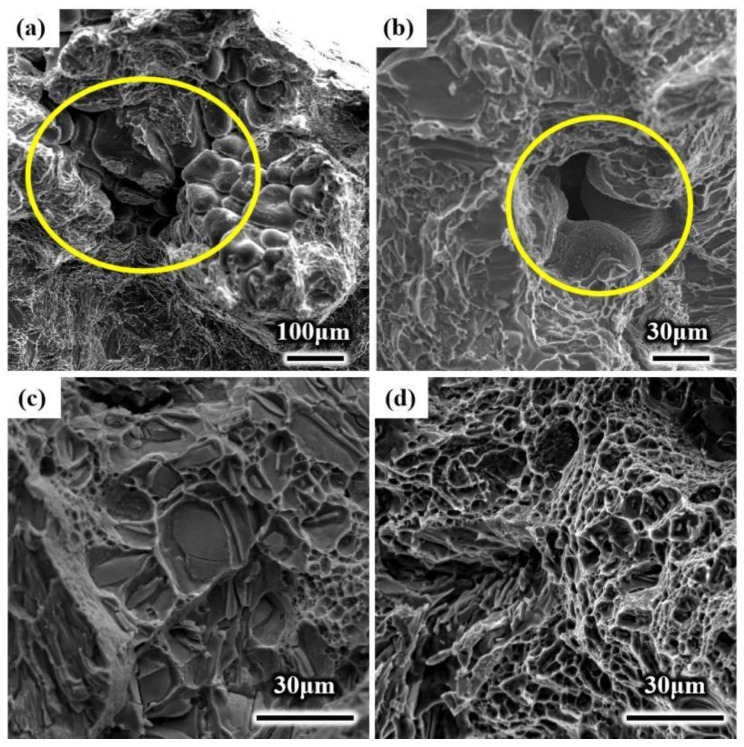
Fracture morphology of (a,c) conventional ingot and (b,d) ultrasonic ingot.
